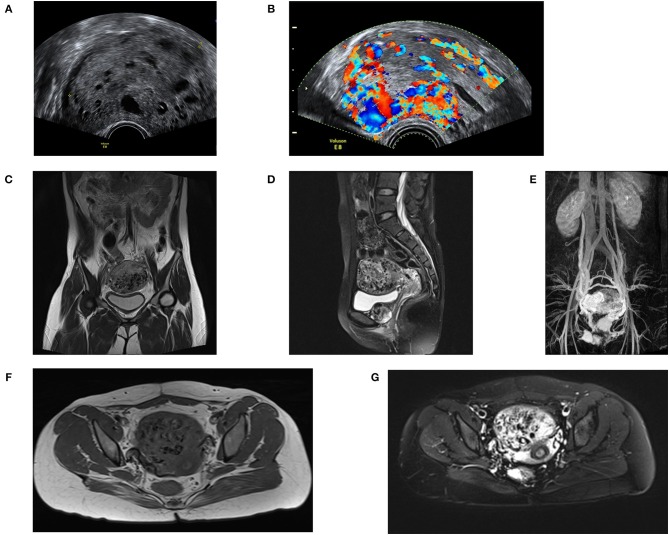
Imaging features of PSTT. (A) Ultrasound feature of PSTT: the mass shows as a mixed mass with intact capsule and presents as echogenic bulk. (B) Ultrasound feature of PSTT: formation of arteriovenous fistula can be observed. (C,D) MRI imaging of sagittal position: PSTT presents as uterine dilatation with short T1 and long T2 signals. (E,F) MRI imaging of axial position. (G) Enhancement imaging: the parauterine artery can be observed.
